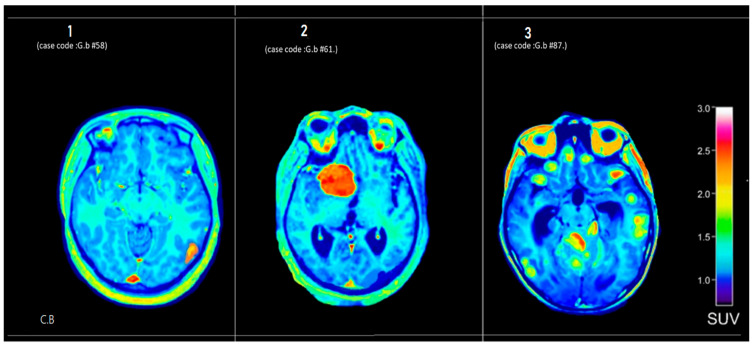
Axial ^18^F-FDG PET brain scans from Center B showing diverse metabolic patterns. Internal case codes (G.b-#58, G.b-#61, G.b-#87) ensure data traceability. Panel 1 (G.b-#58): mild diffuse uptake in skull base (SUV 1.8–2.2), suggesting benign reactive process. Panel 2 (G.b-#61): focal intense uptake in left frontal region (SUV ~3.0), suspicious for aggressive neoplasm or inflammation. Panel 3 (G.b-#87): heterogeneous cerebellar activity (SUV 1.5–2.5), consistent with post-treatment changes or neurodegeneration.
